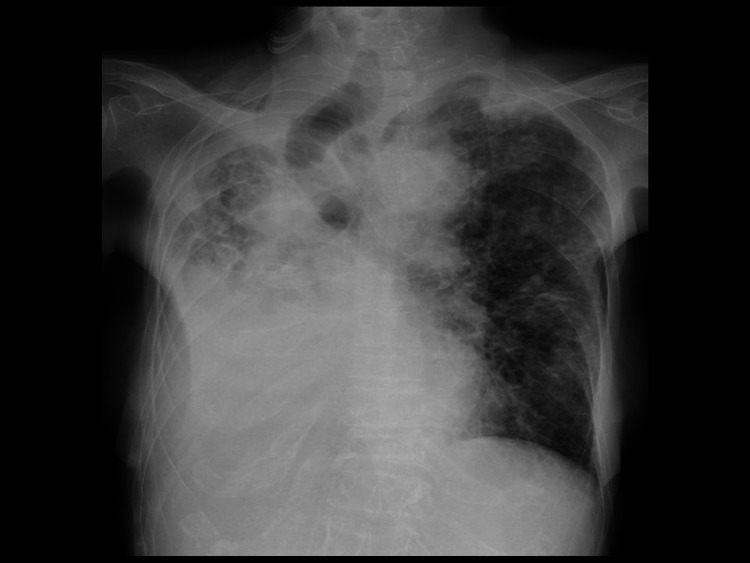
Chest X-ray on admission. Right-sided mediastinal shift and tracheal deviation are observed. The right lung shows atelectasis and infiltrative shadows. The left lung shows ground-glass opacity.
